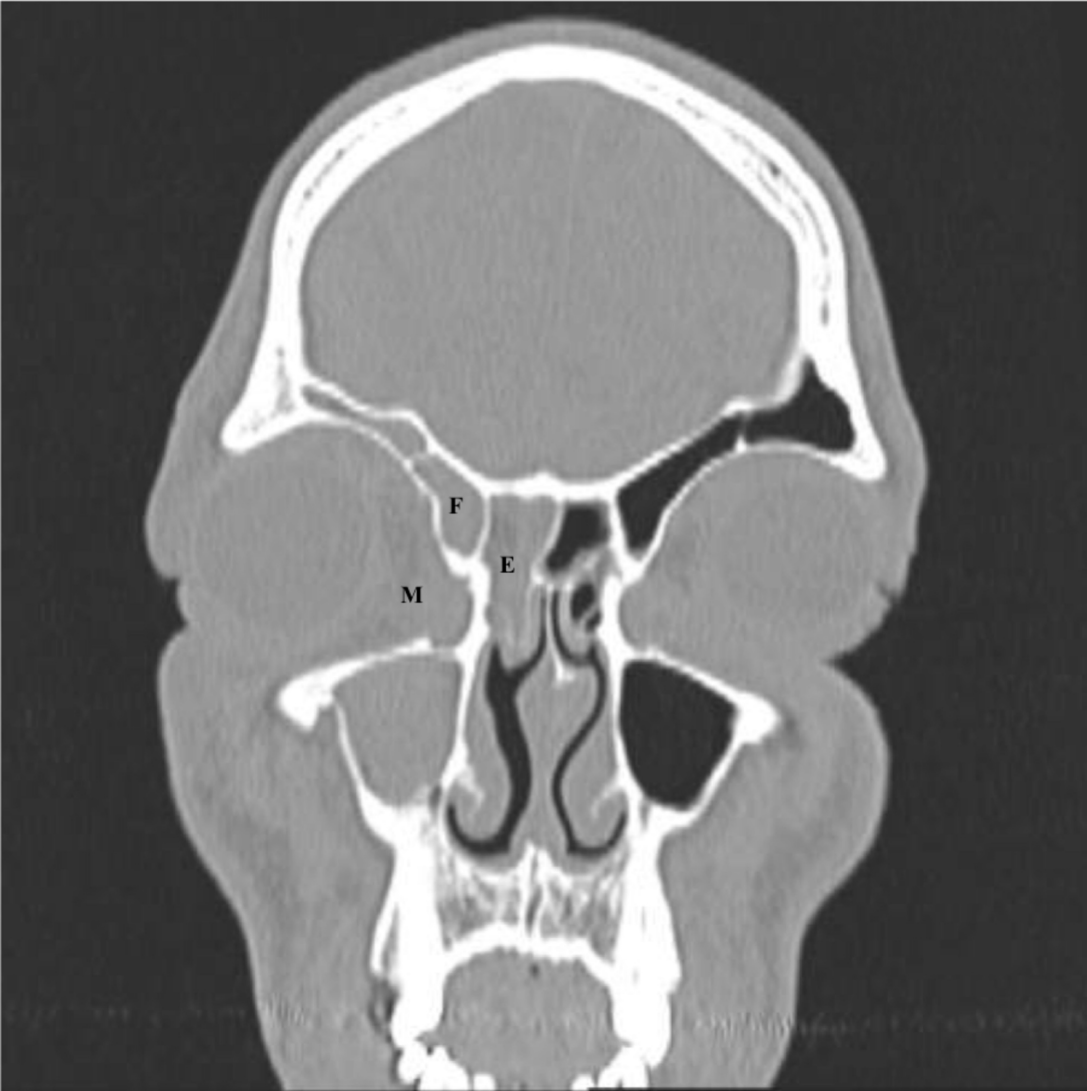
Opacification of the right maxillary, ethmoid, and frontal sinuses on coronal view of computed tomography of the head. M - maxillary, E - ethmoid, F - frontal.
